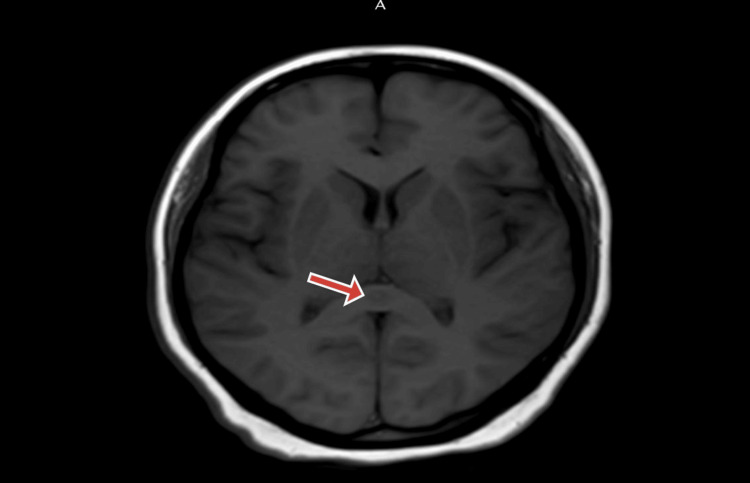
MRI brain axial FLAIR shows an oval area of increased signal intensity in the splenium corpus callosum FLAIR: Fluid-attenuated inversion recovery
